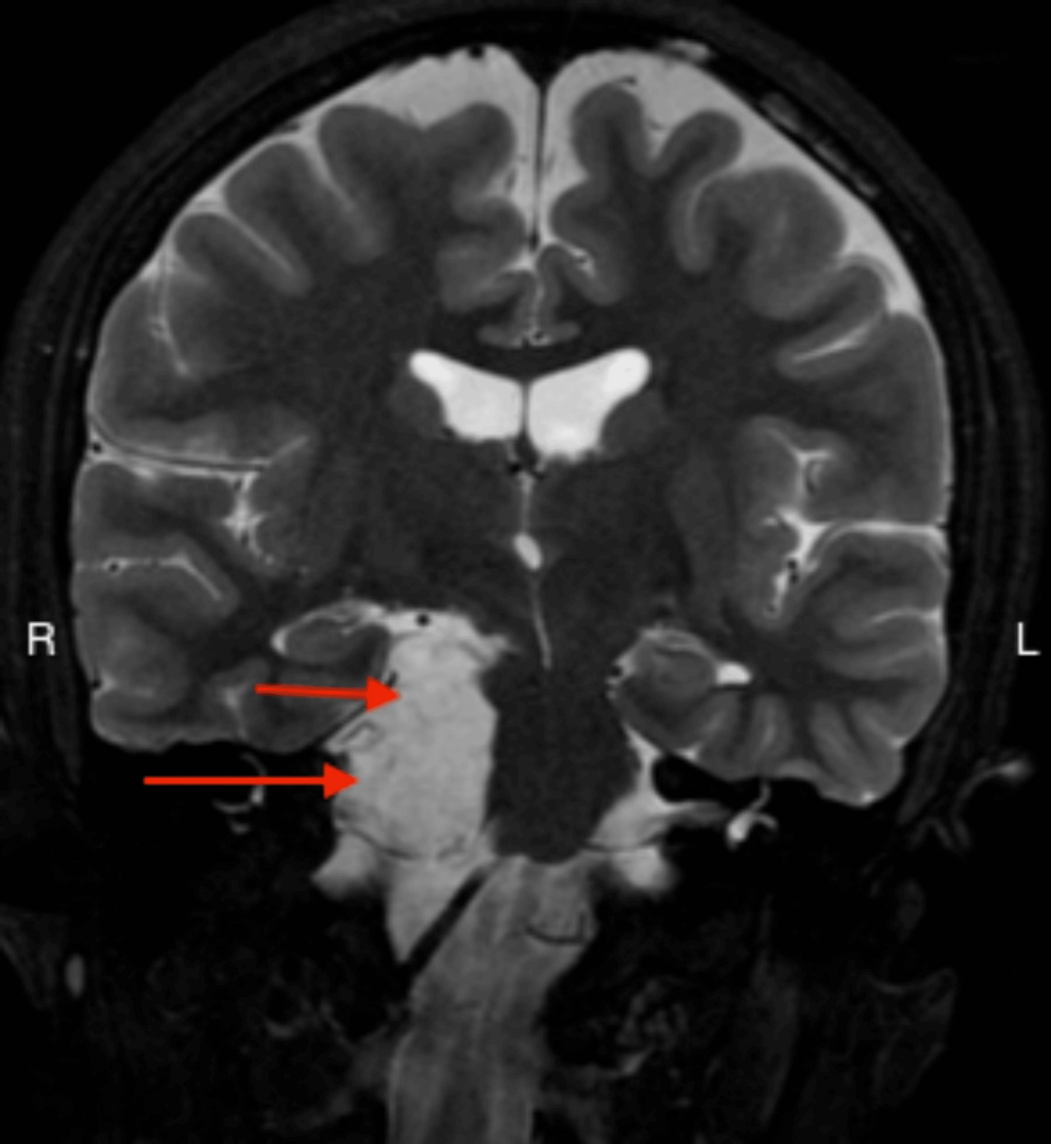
MRI of the brain Coronal T2-weighted MRI image showing hyperintense space-occupying lesion (arrows). MRI: magnetic resonance imaging.
